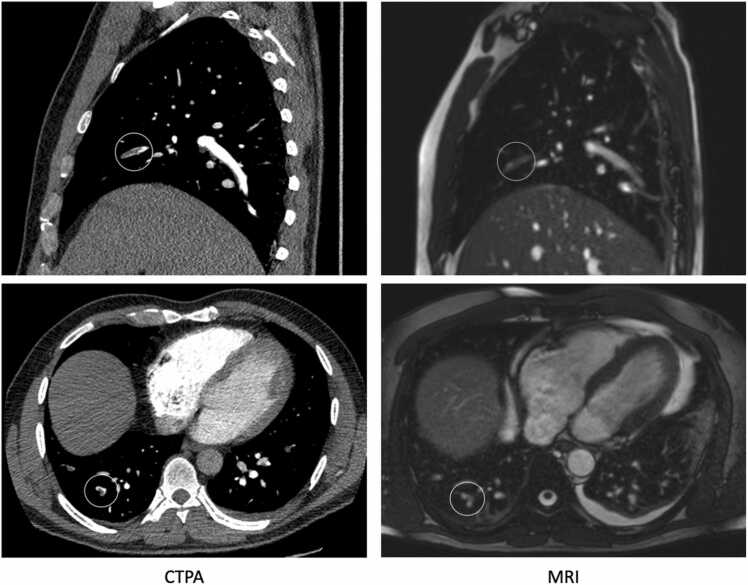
39 years old male, 17 hours between CTPA and MRI. Missed subsegmental emboli in RML and RLL on MRI by R2.
